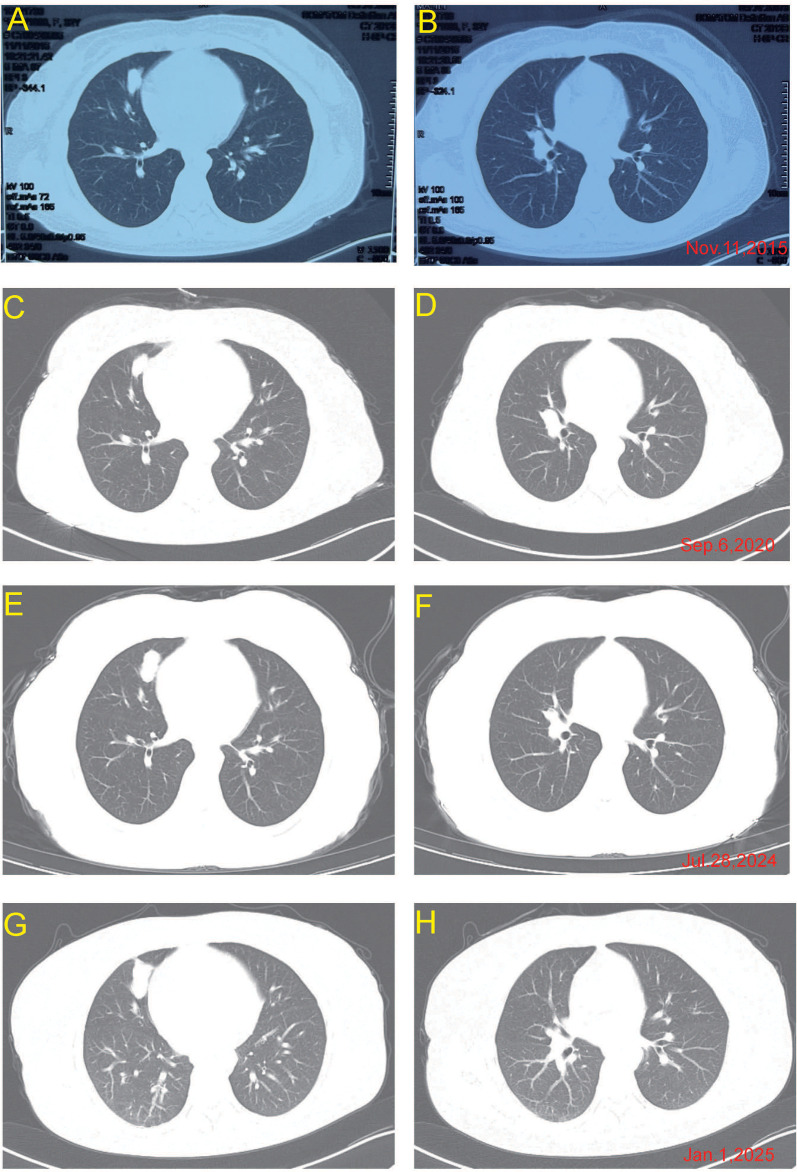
Chest CT follow-up from 2015 to 2025 showing changes in the right middle lobe and hilar lesions. Serial CT images from multiple timepoints demonstrate dynamic changes in the pulmonary lesions over a 10-year period, including post-chemotherapy regression, stable disease, and eventual progression in early 2025. The panels represent: (A, B) Nov 11, 2015; (C, D) Sep 6, 2020; (E, F) Jul 28, 2024; (G, H) Jan 4, 2025.
